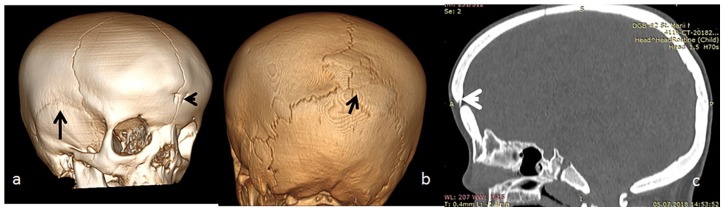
Normal cranium but presence of acute depression of the nasofrontal area and Wormian bones. Three-dimensional (3D) reconstruction of the cranium in a 7-year-old boy with Robinow syndrome, autosomal dominant type, showed persistence of the metopic suture associated with the development of bilateral dimples on the sides of the metopic suture (arrowhead). The squamosal sutures were normal (arrow). The acute depression of the nasal-frontal area is less marked than seen in the autosomal recessive type, similarly a less bulging/bossing of the frontal area. (b) Wormian bones along the lambdoid sutures were notable (arrow). (c) Sagittal 3D-reformatted computed tomography scan showed defective ossification of the frontal bone, which is correlated to the dimples along the metopic suture.
